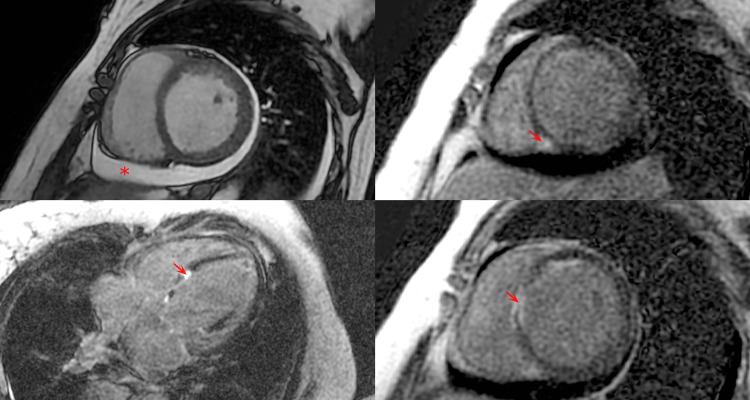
Cardiac MRI performed during the patient’s admission showing areas of late gadolinium enhancement (fibrosis) in the basal septal and medial inferoseptal walls (arrows) in a non-vascular regional distribution pattern and pericardial effusion (asterisk).
